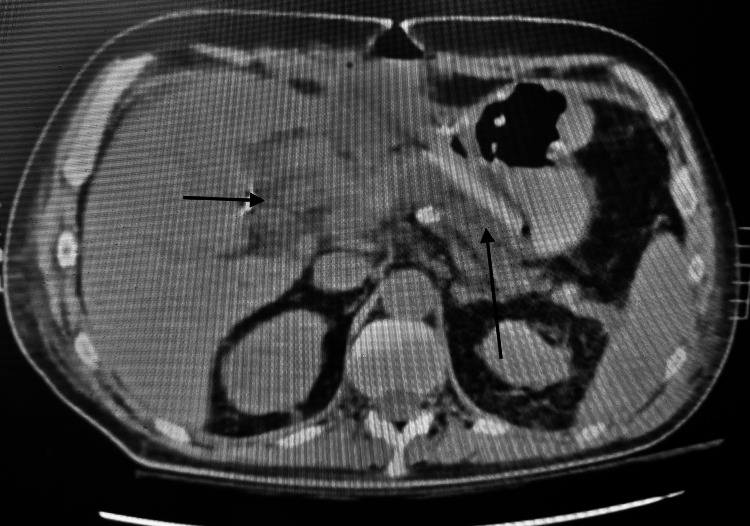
CT view after the removal of coils within the aneurysm (small arrow) and placement of drainage tube next to the lesion (long arrow). CT: computed tomography
